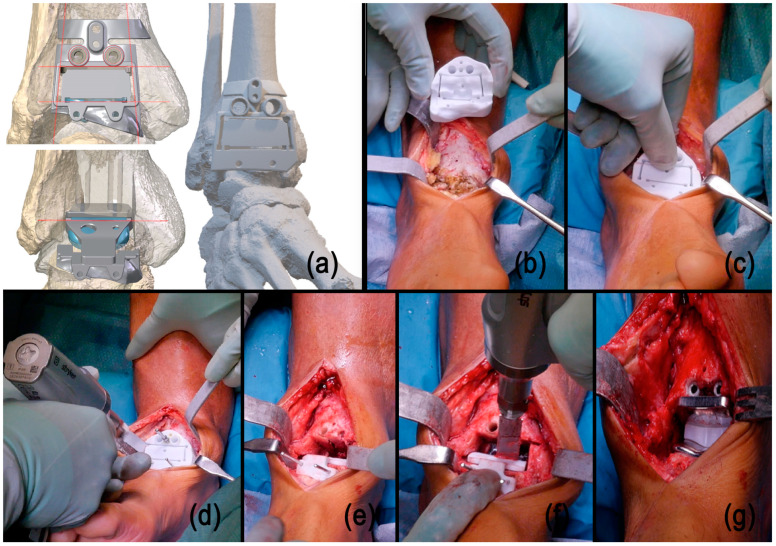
Preoperative CT scans were used to create a three-dimensional model of the ankle, which was used to customize cutting guides (a). The first cutting guide was precisely designed and manufactured to align with the anterior aspect of the joint, including the osteophytes (b,c). Using an oscillating bone saw, talar and tibial resections were carried out through the first cutting guide, which was stabilized with Kirschner wires (d). The second talar cutting guide was then positioned and stabilized using K-wires. This guide facilitated the posterior chamfer resection and included two holes for the preparation of the talar bone pegs (e,f). The final implant was correctly placed into the prepared bone resections (g).
